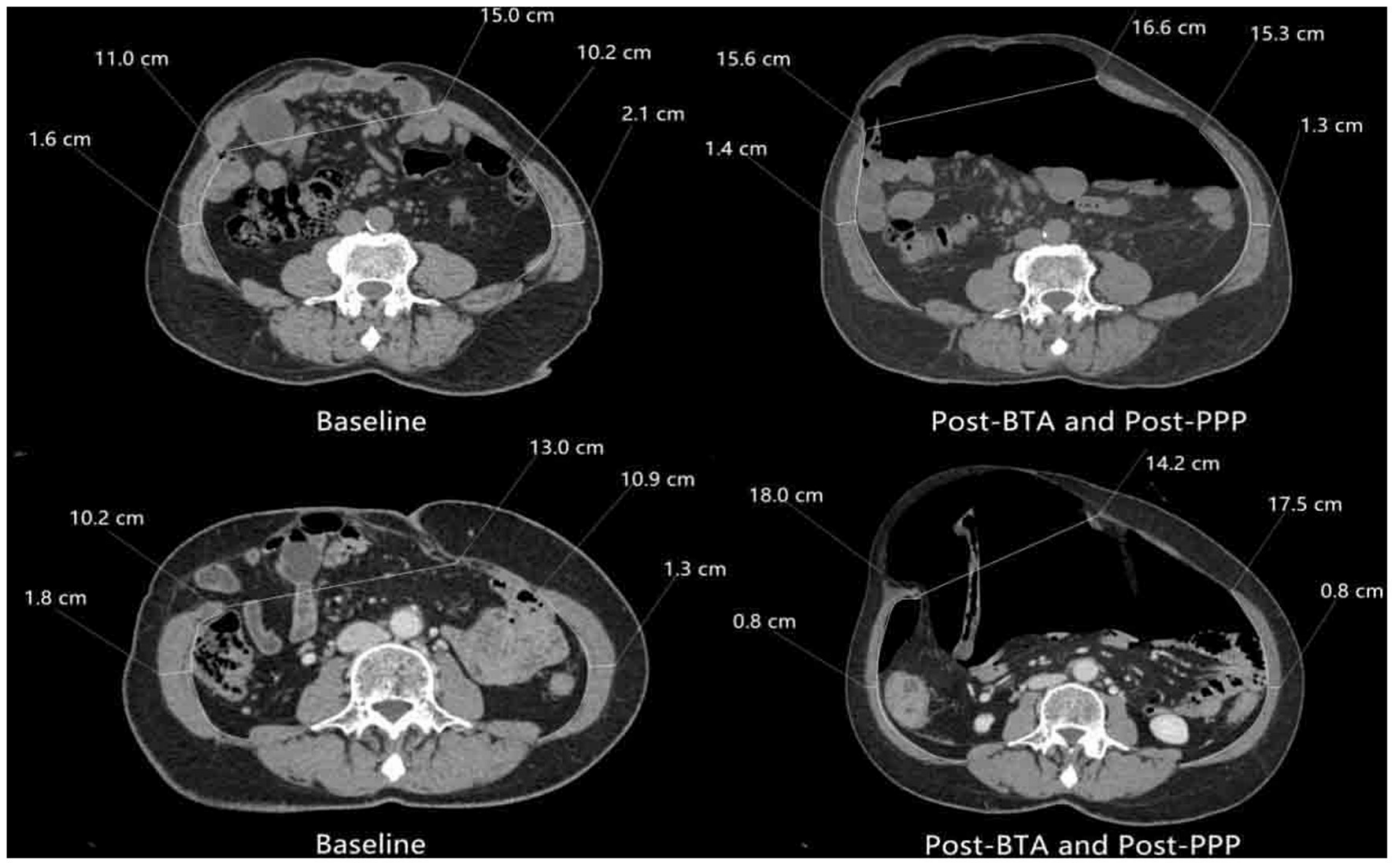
Comparison of preoperative axial computed tomography image before and after preoperative techniques. There is significant elongation and thinning of the lateral abdominal oblique muscles.
